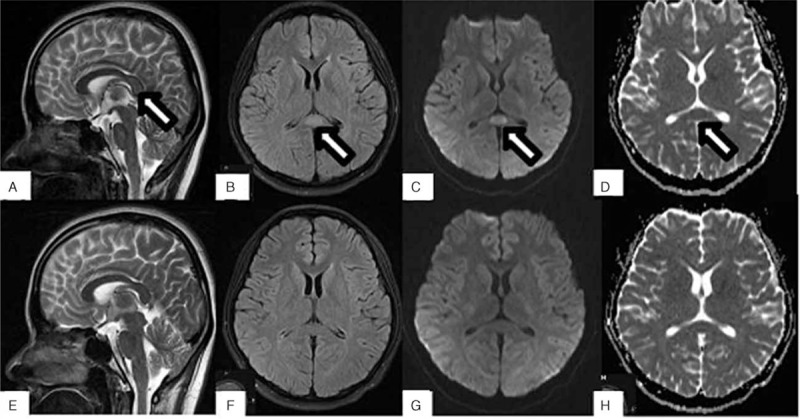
MRI of RESLES, (A) T2WI, (B) FLAIR, (C) DWI, and (D) ADC showed the lesion in SCC (white arrow) at disease onset. (E–H) Correspond to the follow-up images without any evident lesion. ADC = apparent diffusion coefficient, DWI = diffusion-weighted image, FLAIR = fluid attenuated inversion recovery, MRI = magnetic resonance imaging, RESLES = reversible splenial lesion syndrome, T2WI = T2-weighted image.
